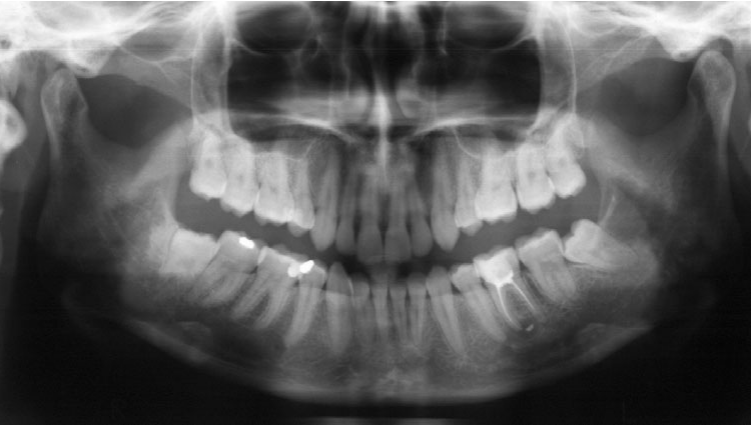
Preoperative panoramic X-ray showing the left lower semi-impacted 3^rd ^molar.
